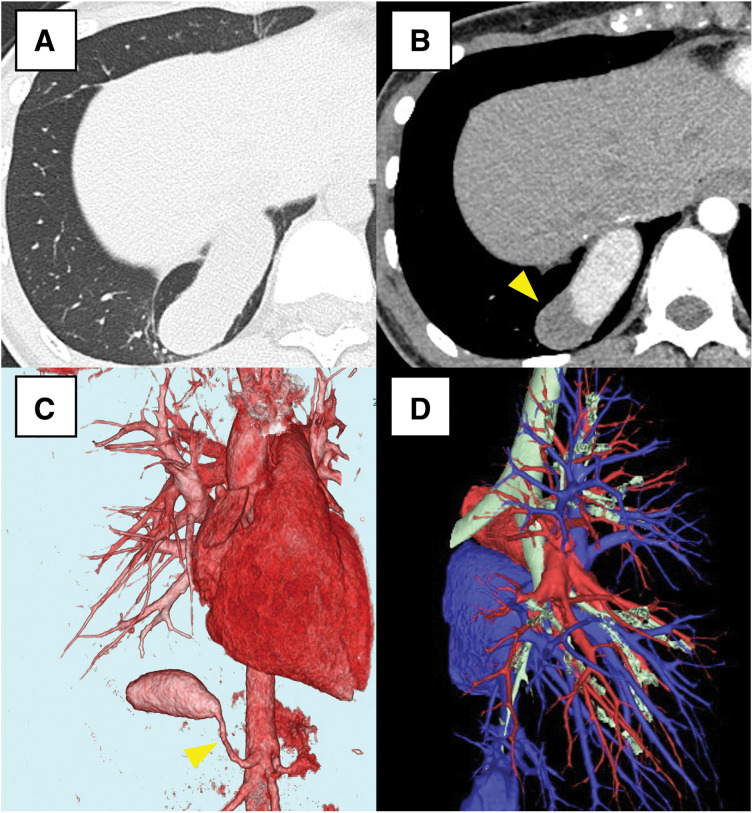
Preoperative contrast-enhanced chest CT scan of Case 1. (A) Lung window shows no congestive changes in the background lung parenchyma. (B) The mediastinal window shows an aberrant artery supplying the right S10, with intraluminal thrombus formation (yellow arrowhead). (C, D) 3D-CT reconstructions show an aberrant artery (yellow arrowhead) originating from the abdominal aorta. Hypoplasia is limited to A10, while the other pulmonary arterial branches are preserved.
